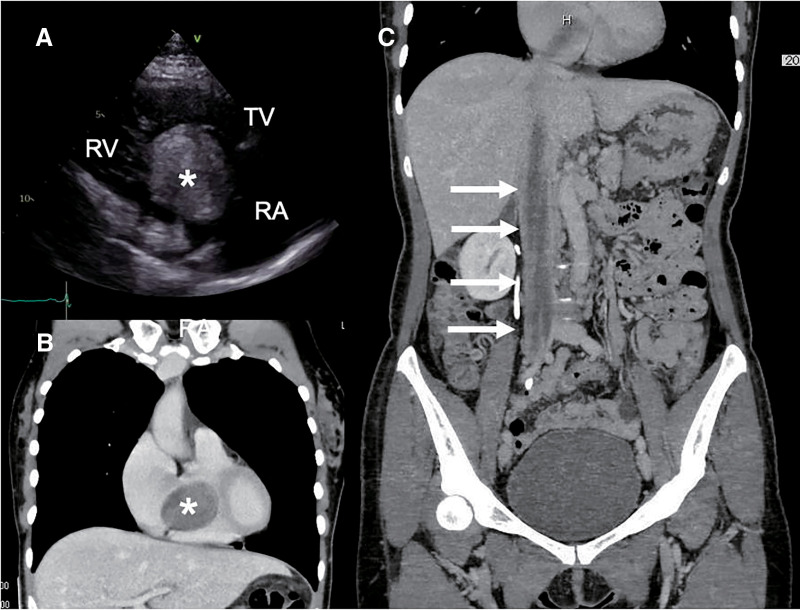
Transthoracic echocardiography and computed tomography. (A) Transthoracic echocardiography revealed the presence of a spherical mass (asterisk), measuring 30 × 30 mm, within the right heart chambers, which was moving across the TV. (B, C) Computed tomography demonstrated a mass (asterisk) extending from the right internal iliac vein to the right heart chamber via inferior vena cava (arrows). RA: right atrium; RV: right ventricle; TV: tricuspid valve
